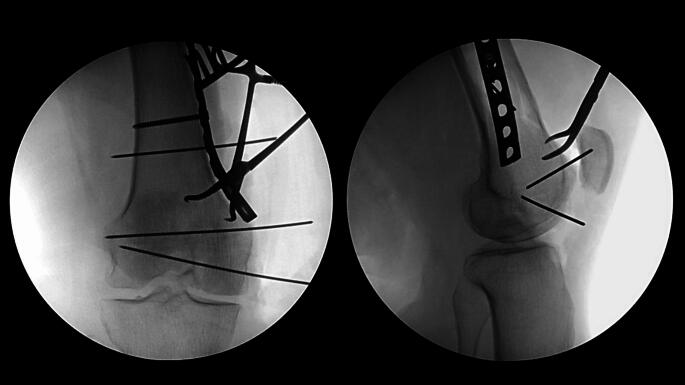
Intraoperative anteroposterior and lateral radiographs displaying buttress plate placement
